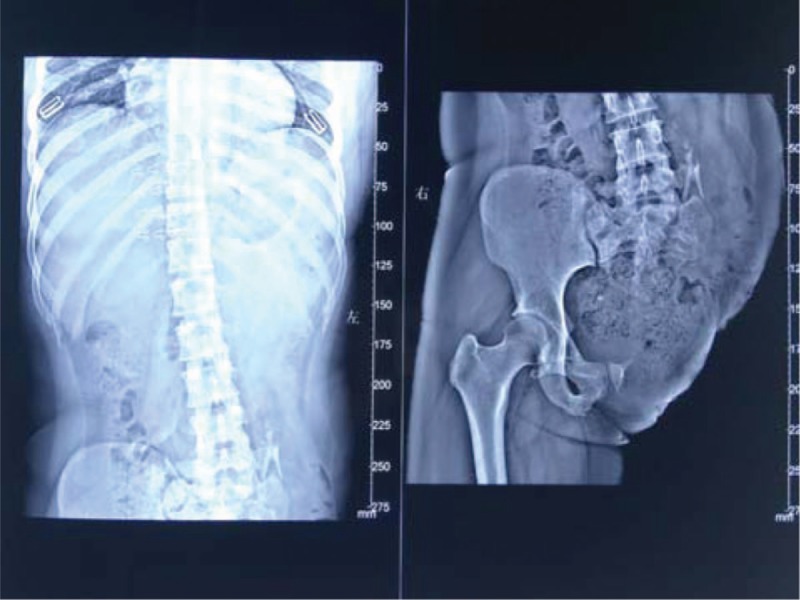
Recent computed tomography scan about 5 years later showing scoliosis of lumbar vertebrae.
